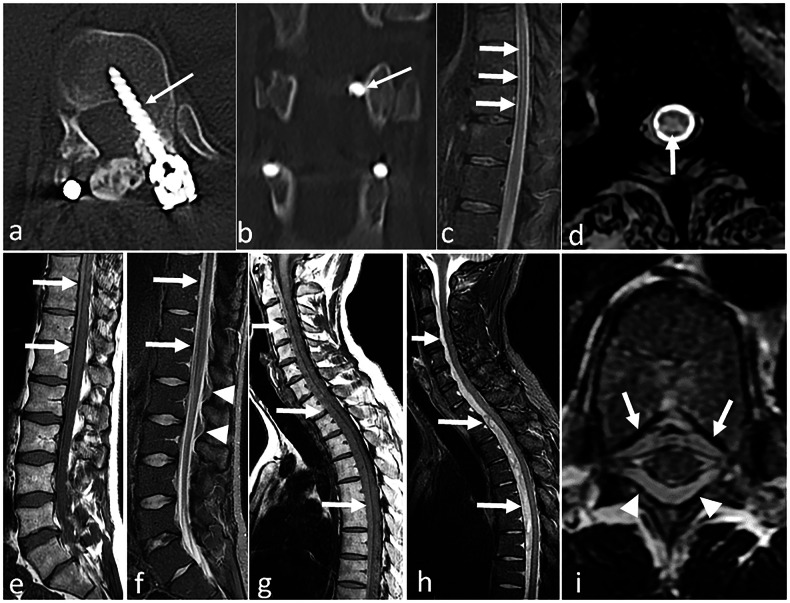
a, b Acute medullary syndrome in a patient waking up from anaesthesia after a thoracolumbar arthrodesis. On CT-scan the left D12 screw is in the spinal canal (arrow) on axial (a) and coronal (b) reconstructions. c, d Acute spinal cord ischaemia in another patient. MRI was performed for a flaccid paraplegia with saddle anaesthesia occurring immediately after herniation treatment. It shows high T2 signal within the cord (arrows) on sagittal (c) and axial (d) views. e–i Epidural haematoma in a third patient. An acute paraplegia was diagnosed following lumbar disc surgery. MRI shows epidural anterior collections that are hyperintense on both T1- and T2-weighted images (arrows). Note that they are difficult to identify on lumbar acquisitions (e, f). Collections appear more clearly on thoracic acquisition on sagittal T1 (g) and fat-suppressed sagittal T2-weighted images (h), as well as on axial T2 images, where a posterior component is also identified (arrowheads) (i)
